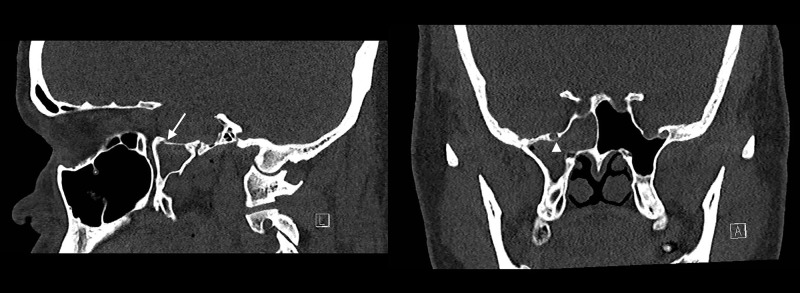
CT pre-contrast panel Computed tomography (CT) shows a discrete focal region of severe bone thinning and probable cortical discontinuity within the anterior, inferior aspect of the right middle cranial fossa just lateral to the foramen rotundum. The contrast material within the middle cranial fossa appears to extend through this defect into the lateral aspect of the right sphenoid sinus.
